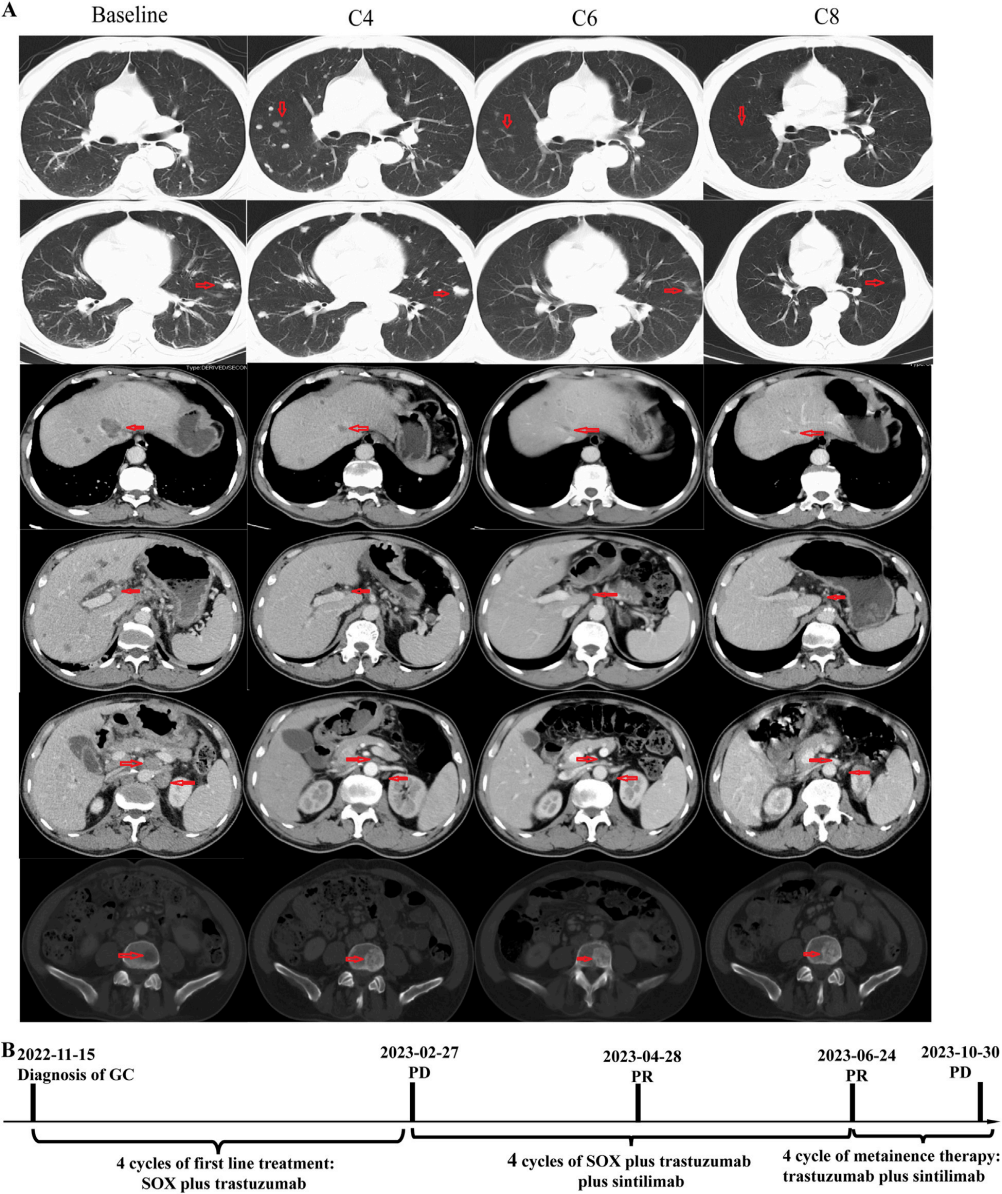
Timeline and representative computed tomography (CT) images. (A) CT images showing patient’s baseline disease, progression after four cycles of SOX plus trastuzumab, and response of PD-1 inhibitor plus SOX plus trastuzumab; (B) Summary of disease course, treatment procedure, and efficacy evaluation of each treatment procedure.
